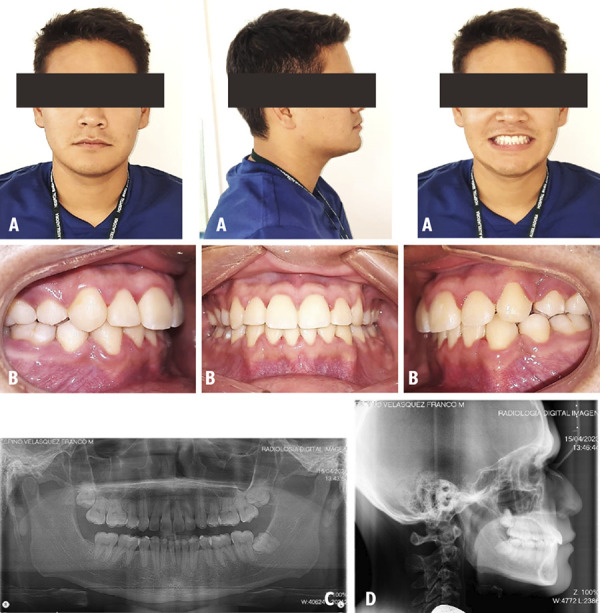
Case 3. Post-treatment records. A: Extraoral photographs. B: Intraoral photographs. C: Panoramic radiograph. D: Cephalometric radiograph.
